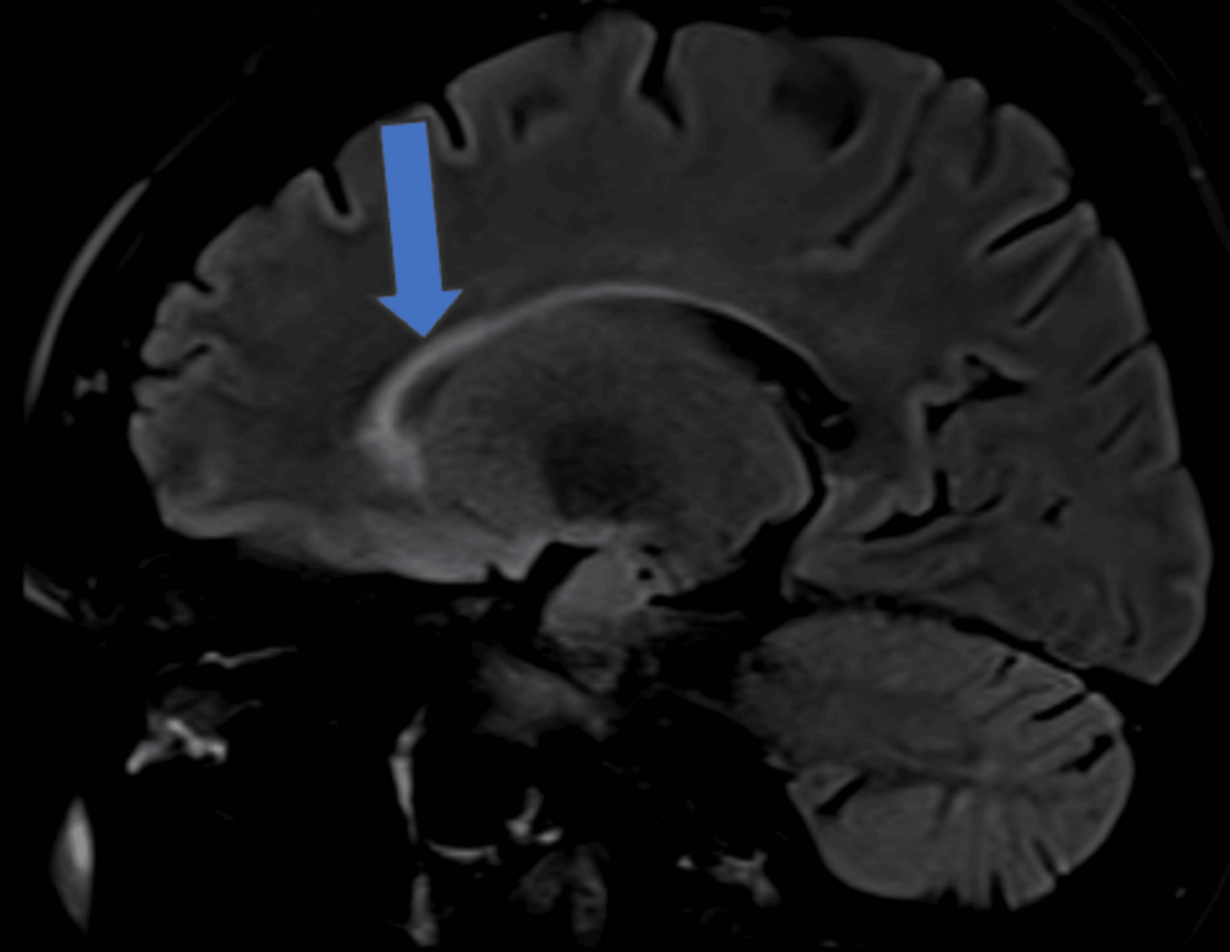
MRI of the brain showing T2 FLAIR hyperintensities in the corpus callosum T2-FLAIR, T2-weighted fluid-attenuated inversion recovery
